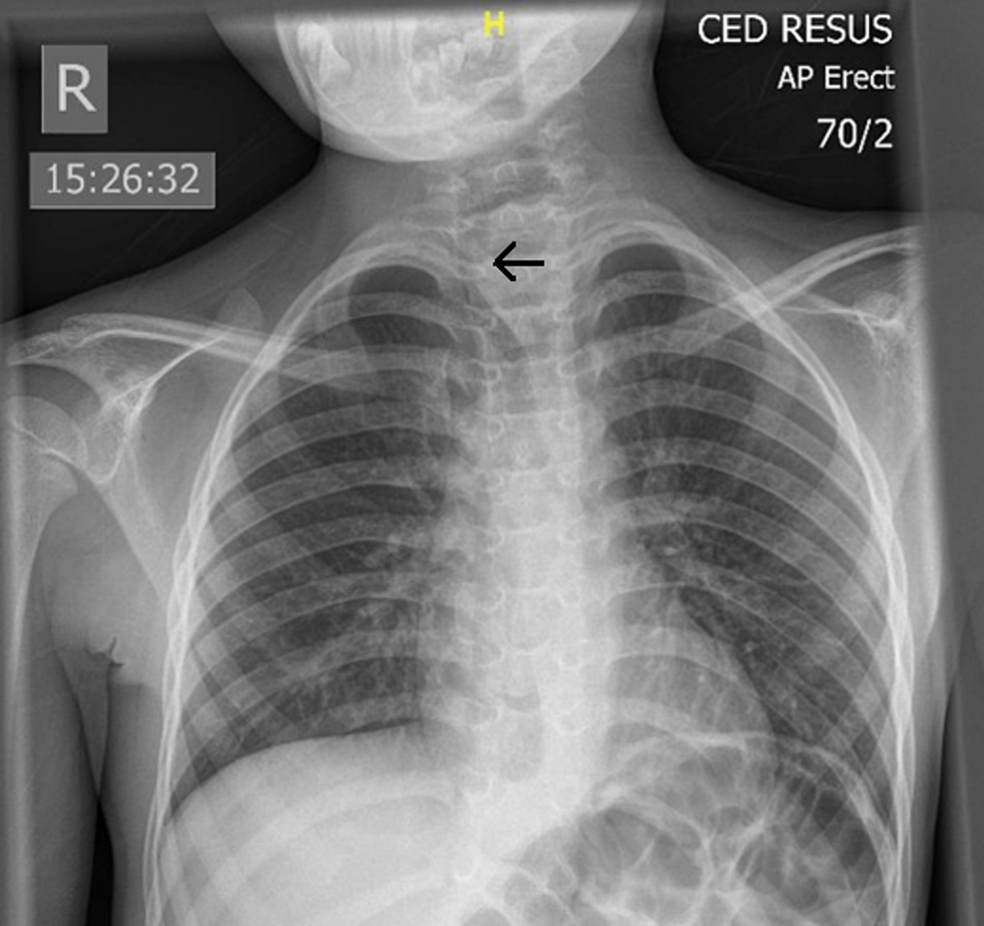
Chest X-ray Admission anterior-posterior erect chest X-ray demonstrating a deviated trachea to the level of the right sternoclavicular joint (arrow) and widened superior mediastinum.
